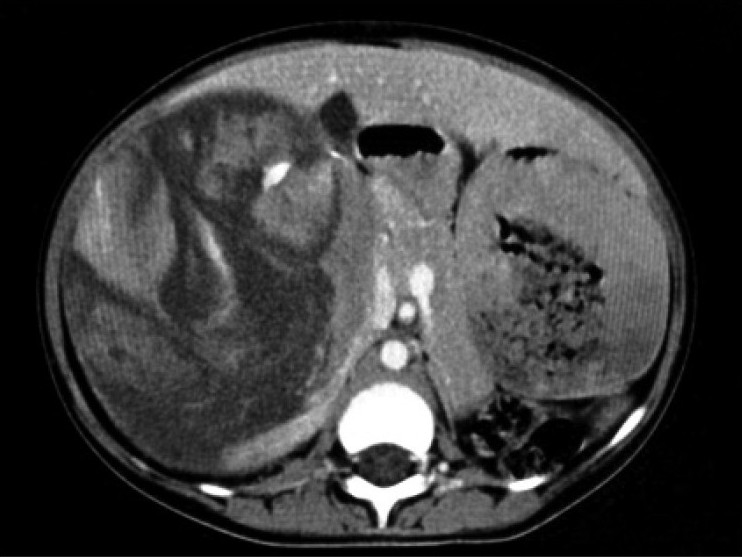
Contrast-enhanced CT scan showing heterogenously enhancing suprarenal lesion with areas of necrosis
